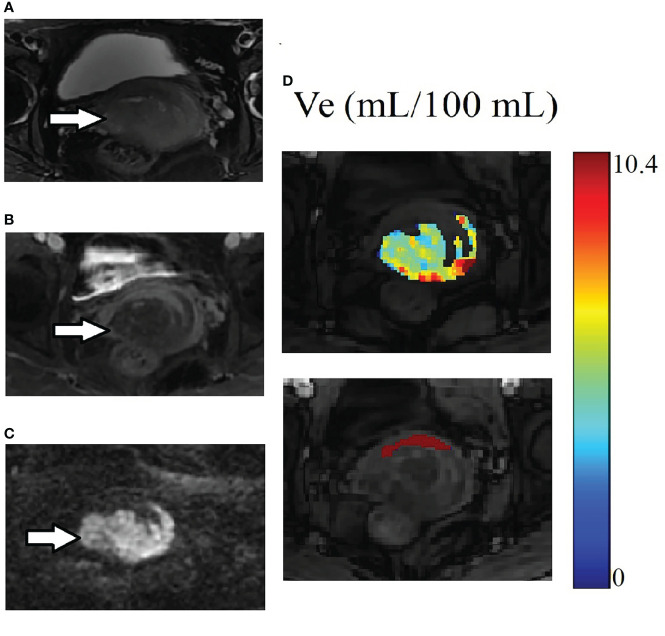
Example of MRI scans for the same patient in Figure 3. (A) Cervix cancer exhibits slightly high signal intensity on axial T2-weighted image. (B) The degree of enhancement for cervix cancer is lower than the normal cervix tissue on delayed contrast imaging. (C) Cervix cancer exhibits high signal intensity on DW image. (D) The parameter Ve maps generated using the ATH model for cancer and the normal tissue ROIs. The upper and lower images are for cancer and the normal tissue ROIs respectively. Parameter Ve value of cervix cancer is significantly smaller than that of normal cervix tissue.
